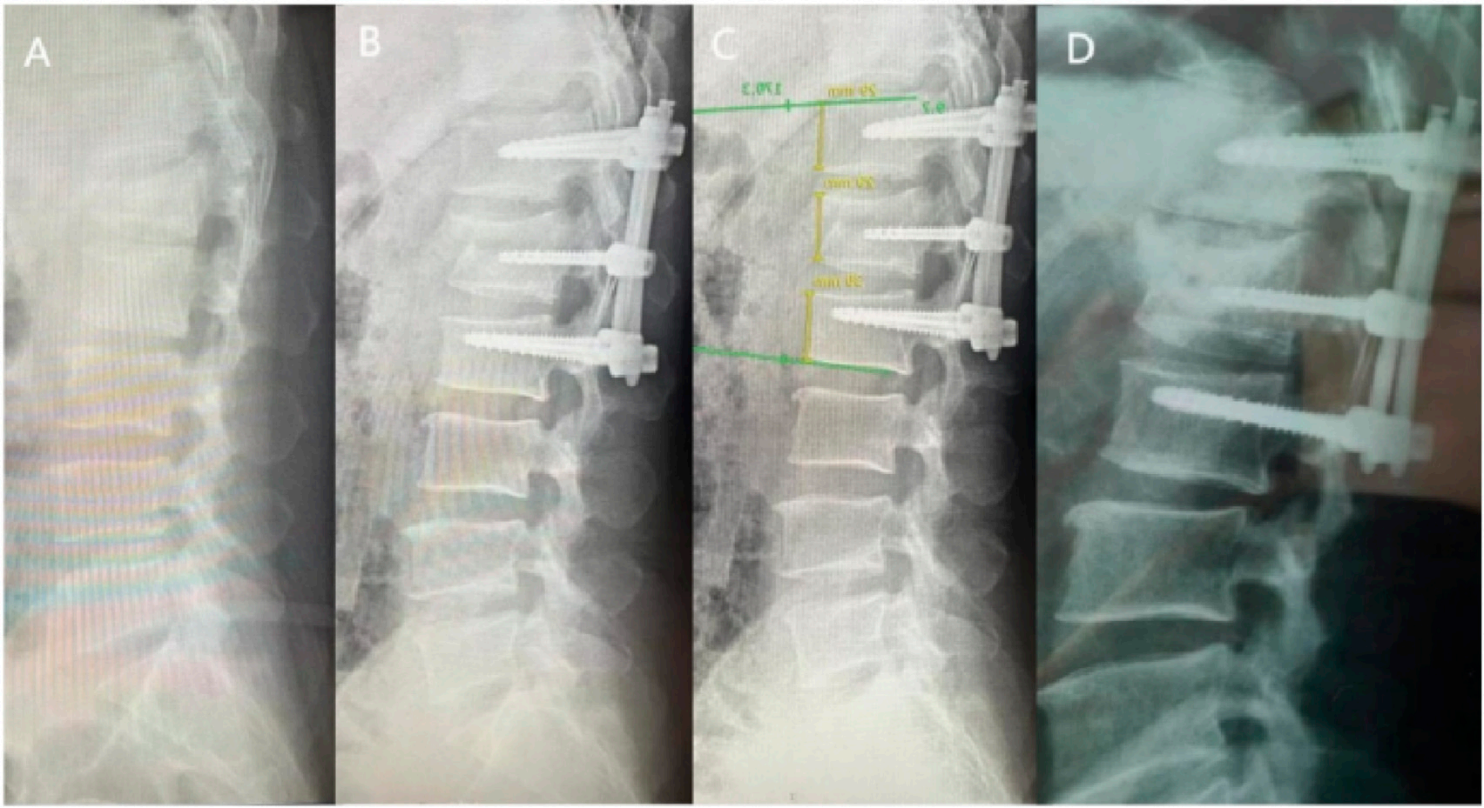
X-rays of a 69-year-old female patient treated with manual percutaneous pedicle screw fixation. (A) Preoperative; (B) 3 days postoperative; (C) 6 months postoperative; (D) 1 year postoperative.
